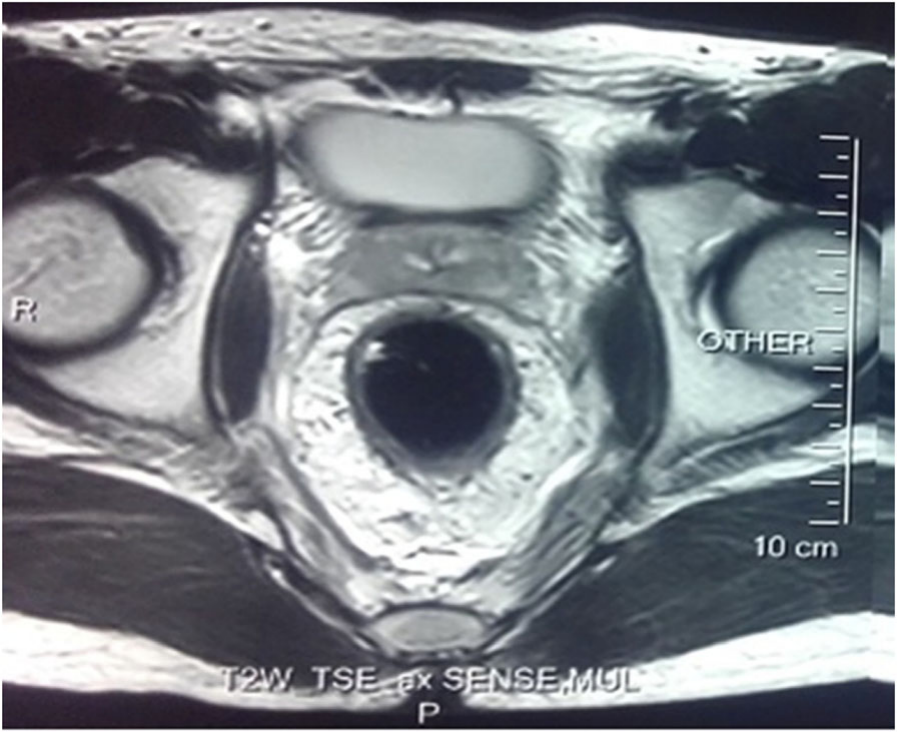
Image showing axial view of a T2-weighted magnetic resonance image of the pelvis, which showed no evidence of recurrence
